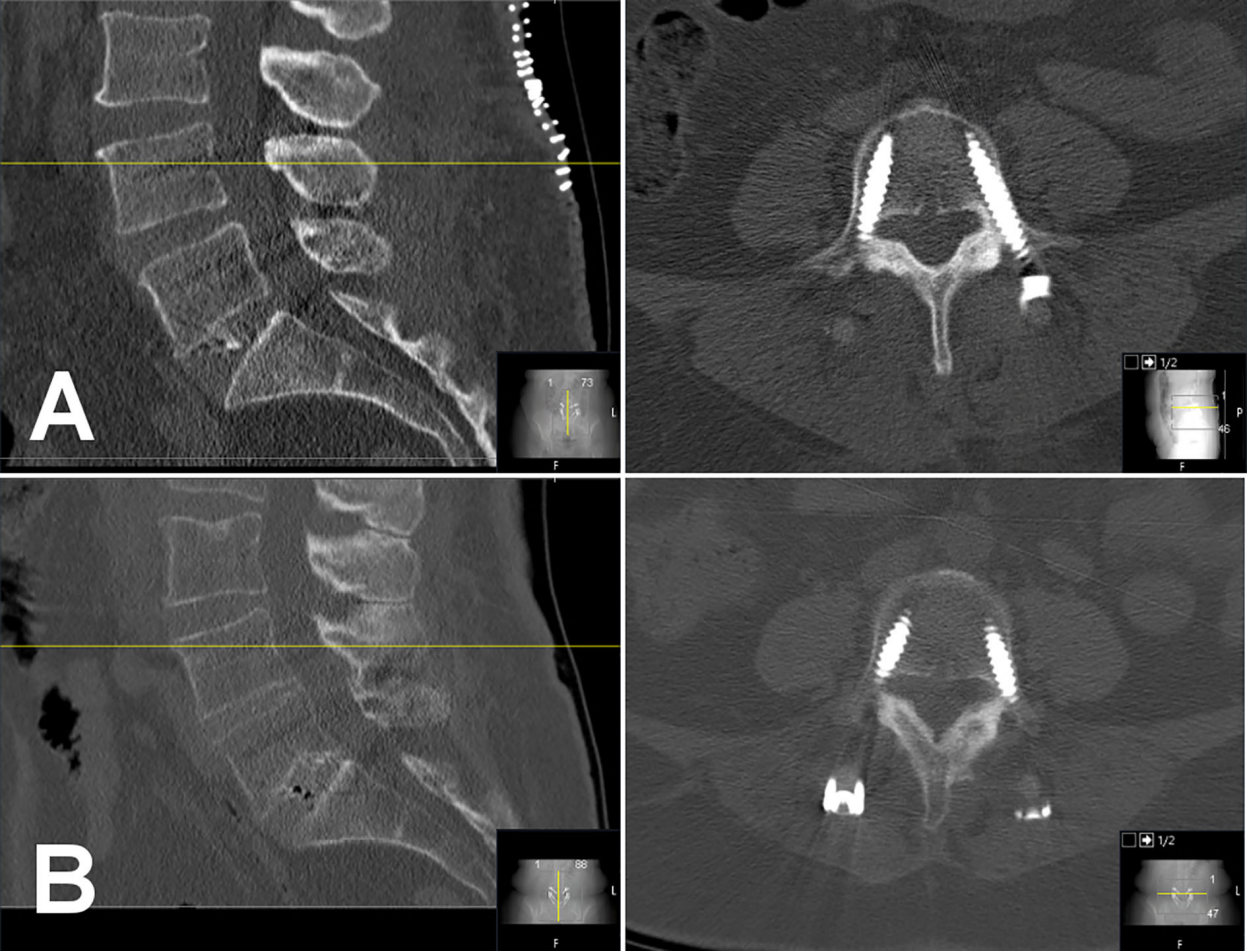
Matched control case of a 48-year-old woman who underwent spinal surgery with semi-rigid instrumentation of levels L4 to S1 for lumbar instability. Opportunistic BMD evaluation yielded normal bone density (BMD = 133.2 mg/cm³). An immediate postoperative CT scans shows all six pedicle screws at L4, L5, and S1 and an intervertebral cage at L5/S1 in place (A). In 12-months survey the patient reported overall benefit from surgery with moderate disability according to Oswestry Disability Index (score 24). A follow-up CT scan more than 7 years after index surgery showed no signs of screw loosening or breakage (screws not fully depicted; B). BMD, bone mineral density.
